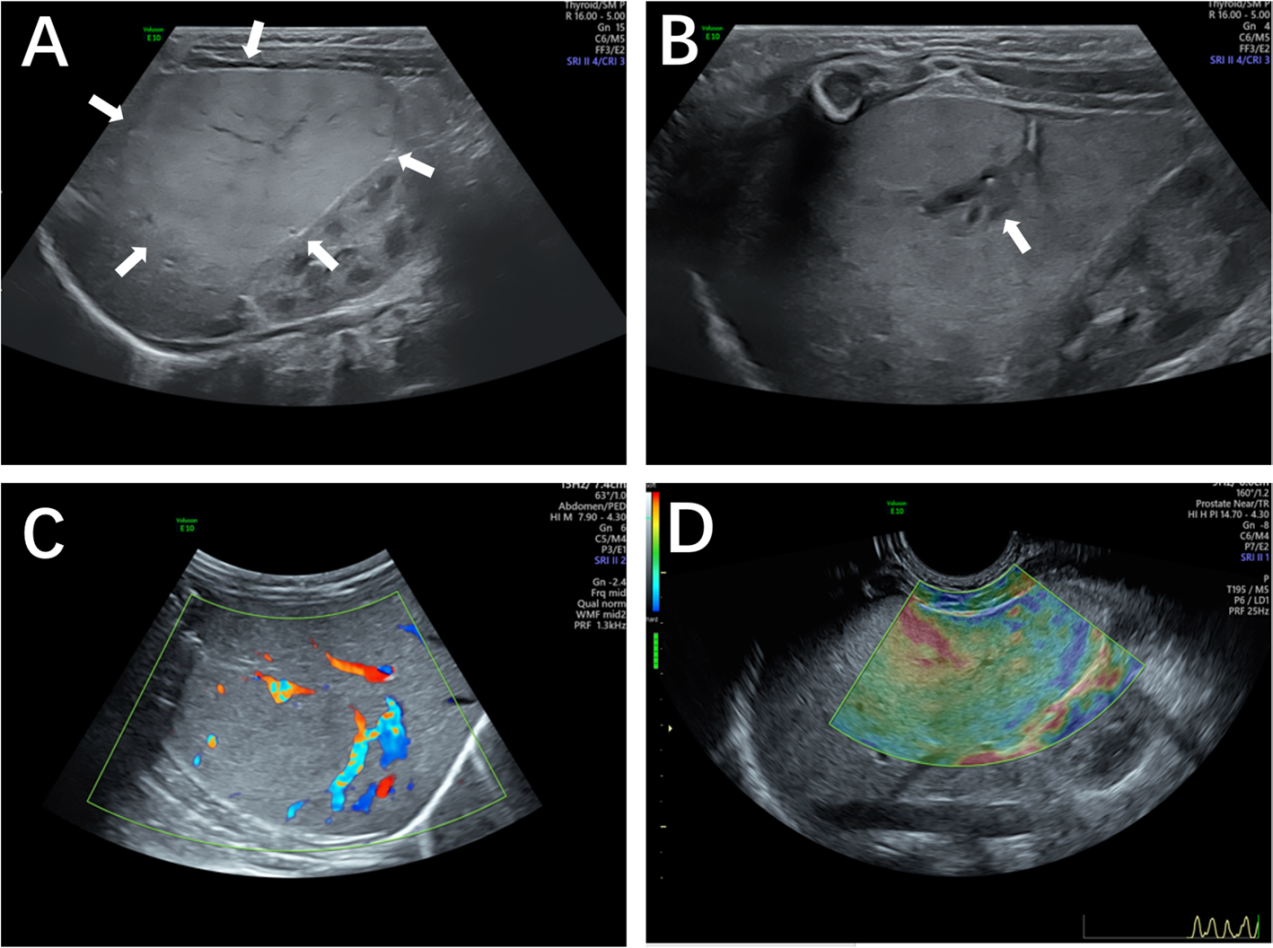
Postnatal ultrasound image of a right lobe hepatic mass. A-B Two-dimensional ultrasound showed a solid hyperechoic mass of approximately 5.5 × 4.5 × 3.7 cm (A arrow) in the infant's right liver, with clear borders, an irregular shape, and a 1.4 × 0.7 cm sheet-like anechoic area with strong light spots (B arrow). C Color doppler showed abundant blood flow signals around and inside the hyperechoic mass of the right liver, and the arterial and venous spectra could be detected. D Elastography revealed a hyperechoic right hepatic mass with low stiffness and compressibility
